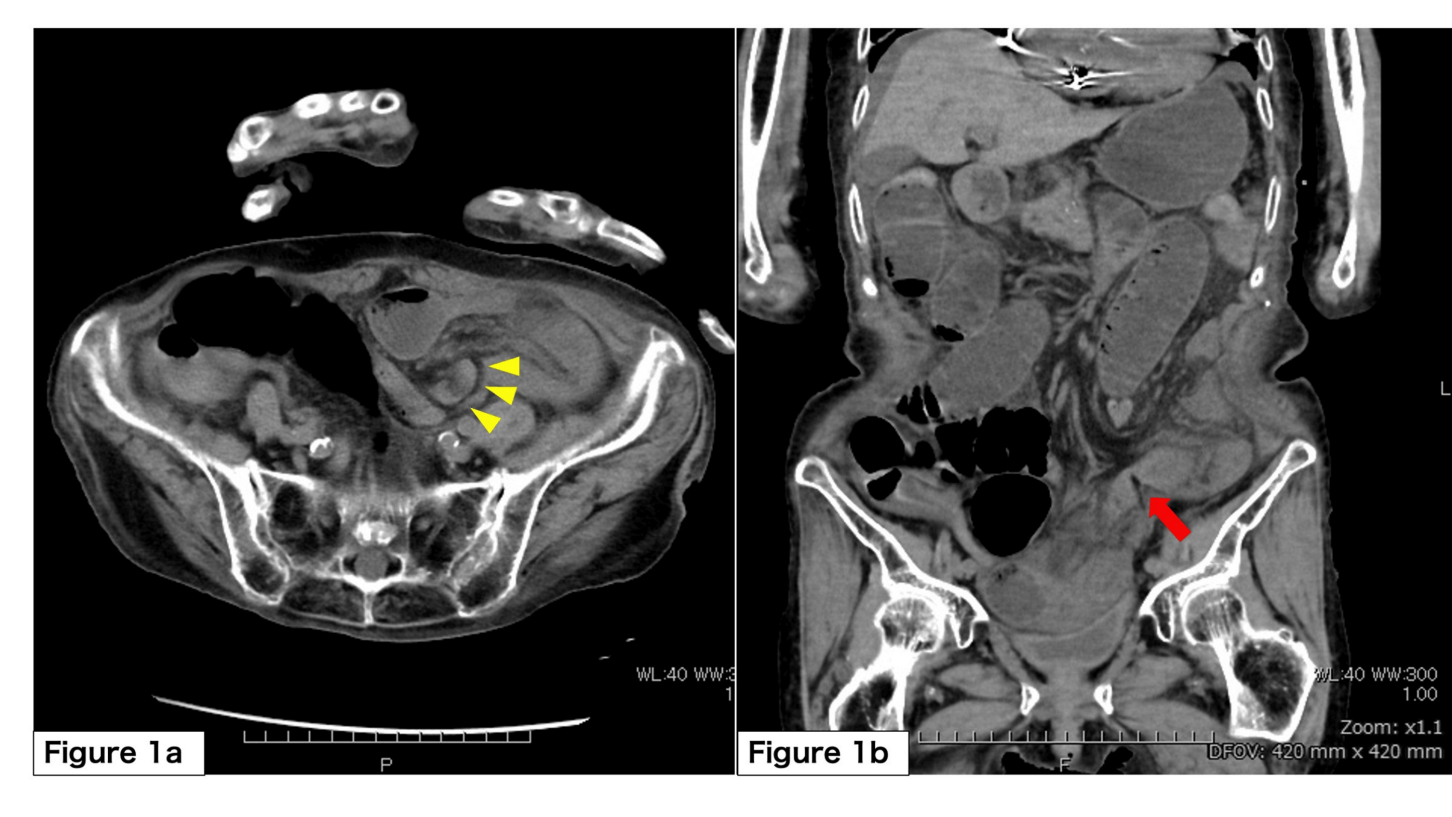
Preoperative abdominal computed tomography (CT) scan (a) Axial view showing dilated small bowel loops and mesenteric edema; (b) Coronal view demonstrating the transition point in the left lower abdomen.
